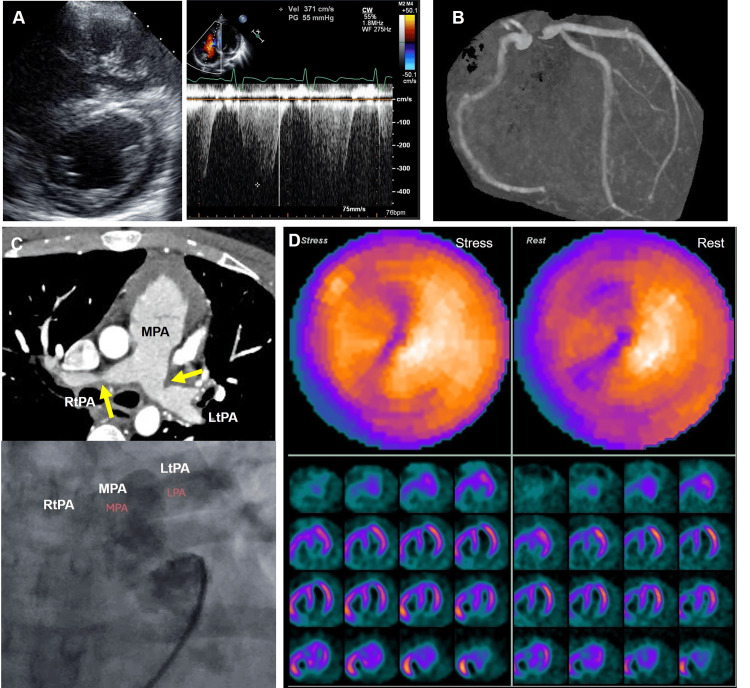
Cardiac Images at 20 years of age A: Echocardiogram at the age of 20 years. Mild pulmonary hypertension was indicated by the shape of the interventricular septum (left panel) and velocity of tricuspid valve regurgitation (right panel). The peak velocity of tricuspid regurgitation was 3.7 m/s, which corresponds to a pressure gradient of 55 mmHg. B: No stenotic lesion is identified in the coronary arteries on computed tomography angiography. C. The computed tomography angiography revealed bilateral pulmonary stenosis (yellow arrows, upper panel). The catheter angiogram revealed antegrade blood flow to the bilateral pulmonary arteries (lower panel). D: Nuclear cardiac perfusion imaging is shown. Neither stressed nor resting images indicated perfusion defects. Abbreviations: LtPA, left pulmonary artery; MPA, main pulmonary artery; RtPA, right pulmonary artery.
